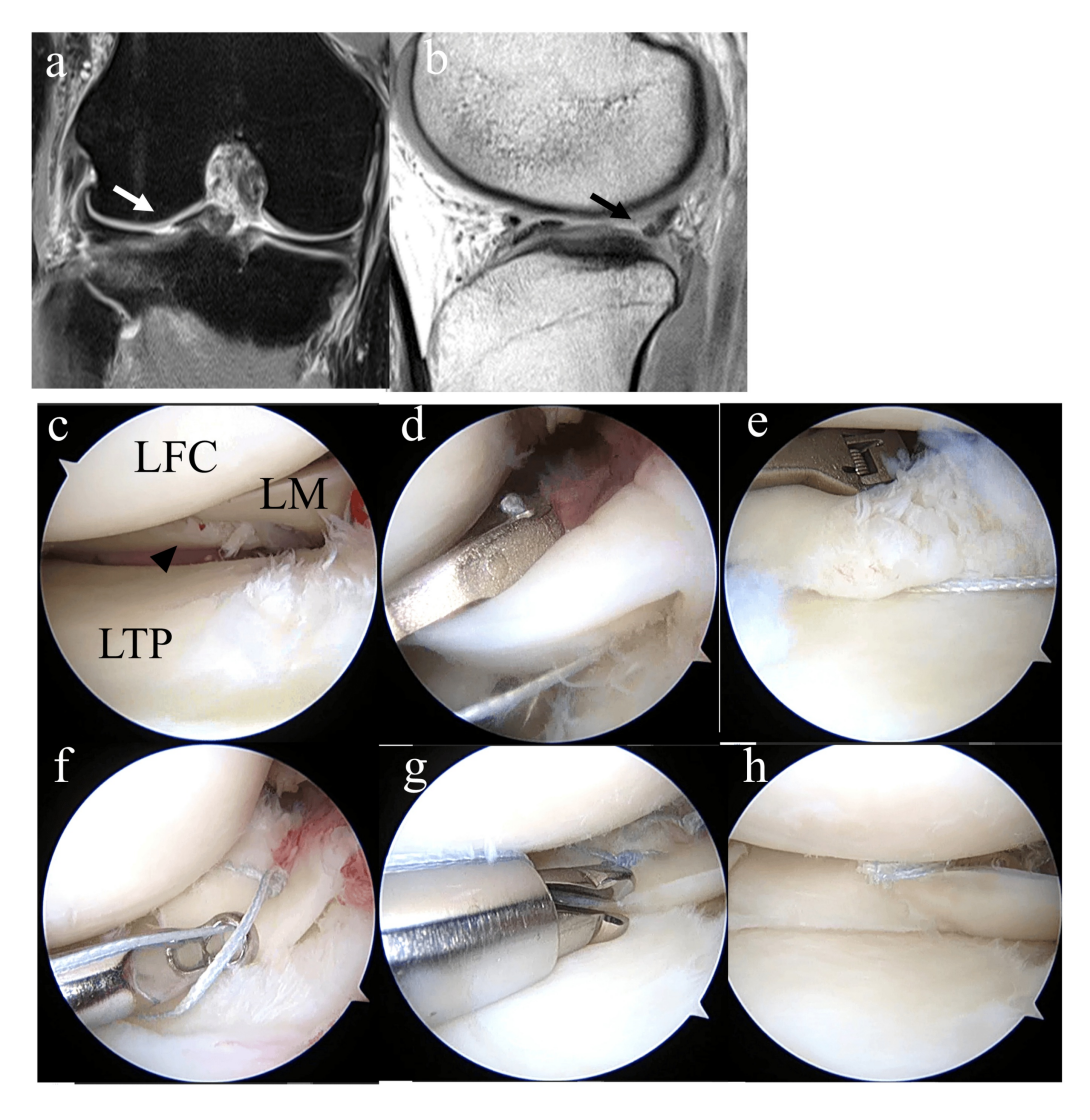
Representative case 2 (a) Preoperative MR image showing a vertical linear defect in the coronal plane to indicate LMPRT (white arrow). (b) Truncated triangle sign (arrow). (c-k) Arthroscopic findings. (c) Type2c LMPRT (arrowhead). (d) One end of a 2-0 FiberWire is placed at one side of the tear using the Knee Scorpion Suture Passer. (e) Another end of the 2-0 FiberWire is placed at another side of the tear to form a horizontal mattress suture. (f) The suture is tied with a sliding knot. (g) The knot is cut with a suture cutter. (h) After two horizontal mattress sutures. MR: Magnetic resonance; ACL: Anterior cruciate ligament; LMPRT: Lateral meniscus posterior root tear; LM: Lateral meniscus; LFC: Lateral femoral condyle; LTP: Lateral tibial plateau.
